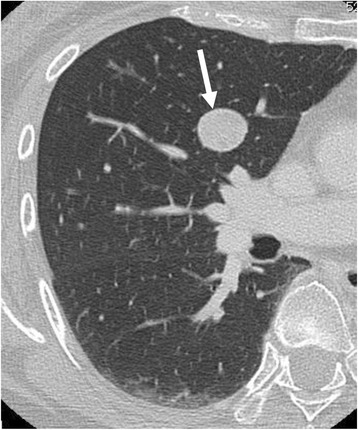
Chest computed tomography (CT) scan obtained 5 years after the first surgery. A contrast-enhanced CT scan of the chest reveals a well-defined large solitary pulmonary nodule (*arrow*) in the right middle lobe
